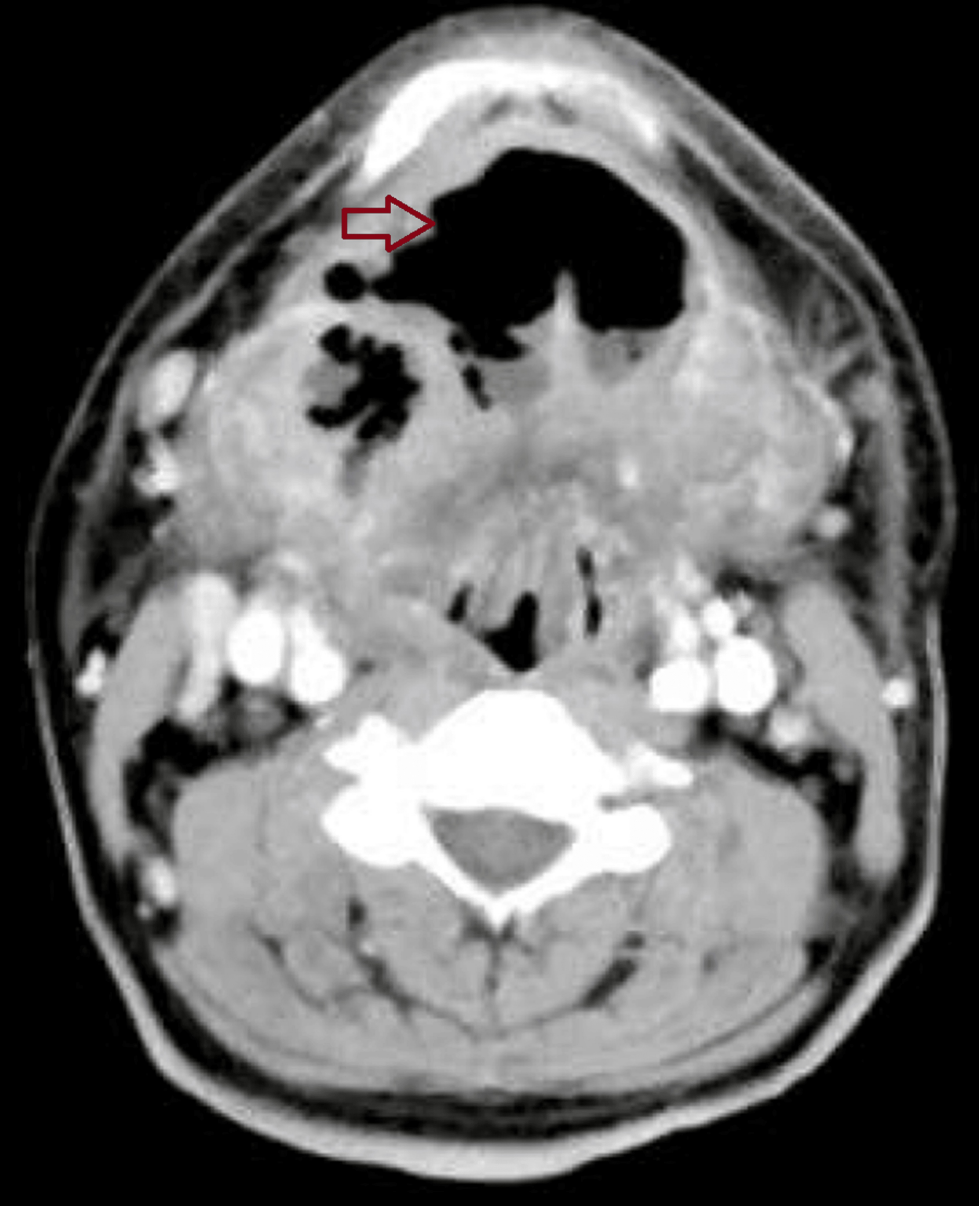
Contrast CT Neck Thickening and increased attenuation diffusely on the floor of the mouth, submandibular space. Associated gas collection on the floor of the mouth and bilateral cervical lymphadenopathy. CT=computed tomography
